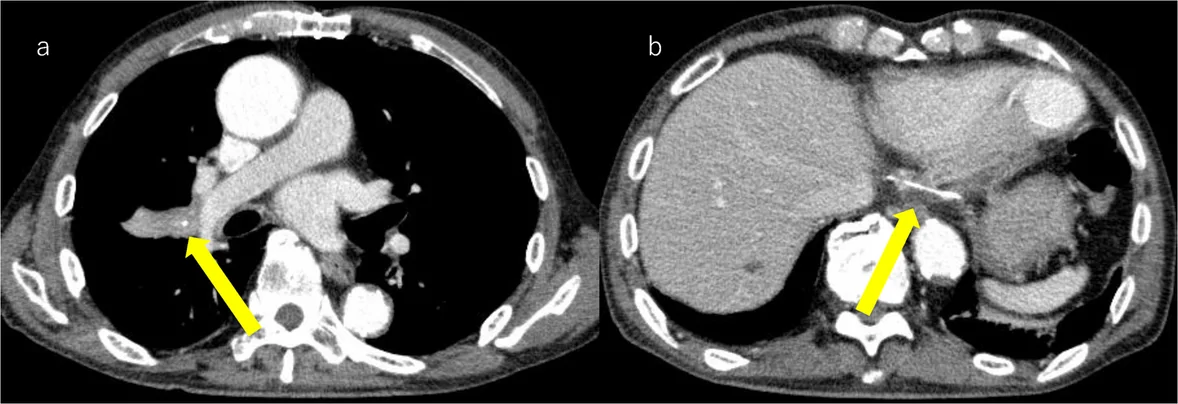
a Contrast-enhanced axial chest computed tomography showing a linear high-density structure in the right hilum (arrow). b Continuity of the same structure extending toward the inferior aspect of the heart (arrow)
